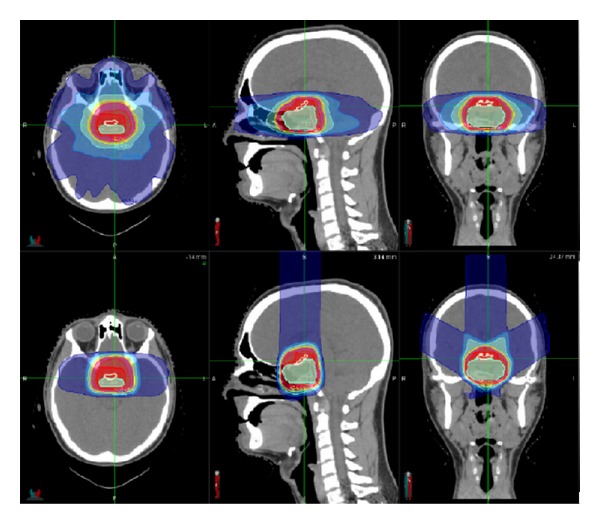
Proton radiation dose distribution. Patient with a tumor in the pituitary gland, receiving proton radiation to the tumor (innermost cyan shaded area). Upper three images show the radiation dose distribution from a photon radiation treatment plan using multiple converging beams on axial CT image (left upper), sagittal CT image (middle upper), and coronal CT image (right upper). Lower three images show the radiation dose distribution from a proton radiation treatment plan using three proton beams on axial CT image (left lower), sagittal CT image (middle lower), and coronal CT image (right lower). For all images, innermost red line = everything inside the line is receiving at least 100% of the full prescribed radiation dose, and outermost blue line = everything inside the line is receiving at least 20% of the full prescribed radiation dose. Note that the high radiation dose region (inside the red line) looks similar between the photon and proton plan, but the lower radiation dose region (inside the blue line) is much smaller with proton radiation than photon radiation.
